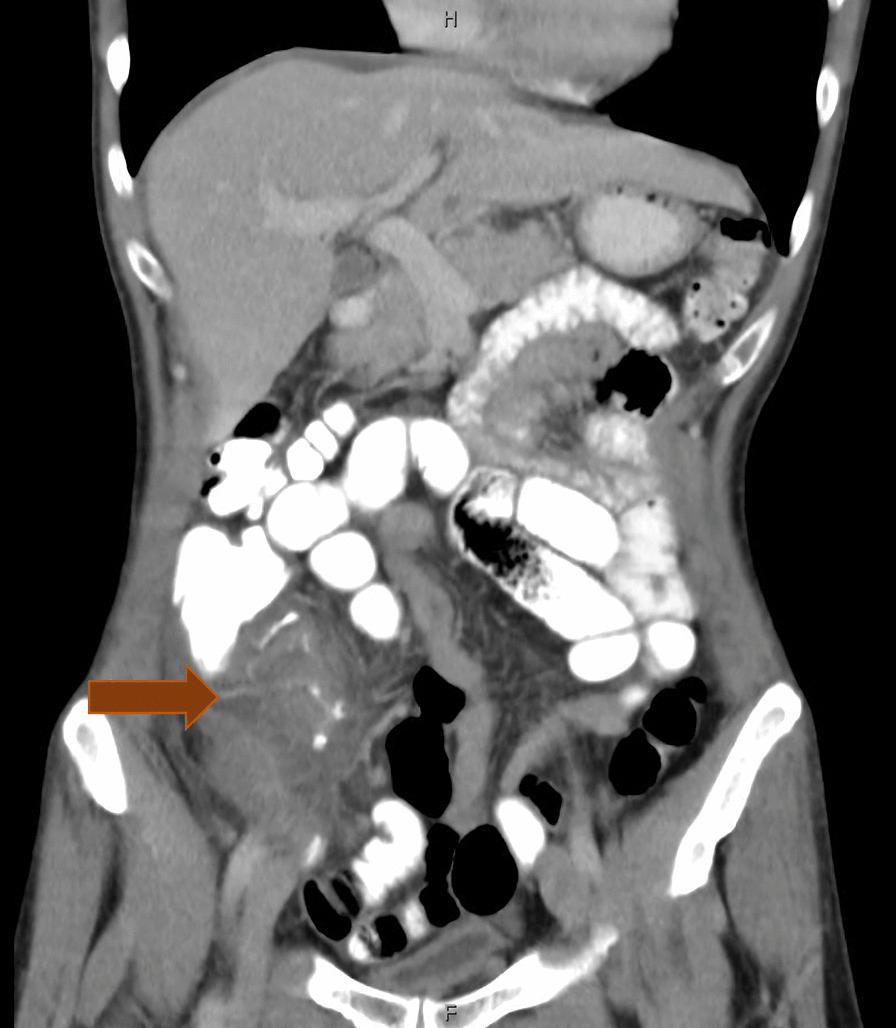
Contrast-enhanced coronar CT scan of the abdomen and pelvis shows mucinous mass of the coecum and appendix (red arrow)
